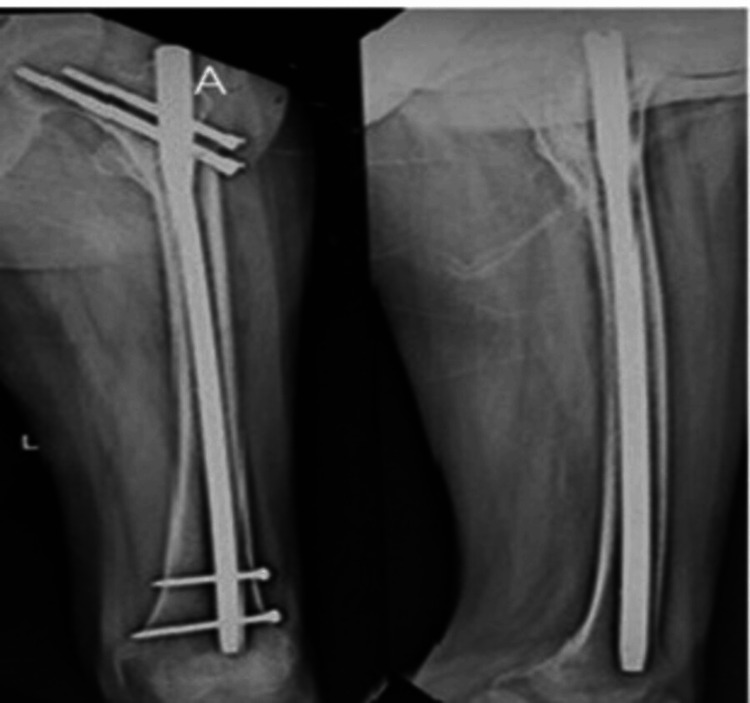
Six-month follow-up radiograph of the same patient The patient, whose radiograph is shown in Figure 1, underwent AP and lateral radiographic imaging of the left hip joint and femur six months postoperatively, showing radiological union of the intertrochanteric fracture. AP: anteroposterior.
